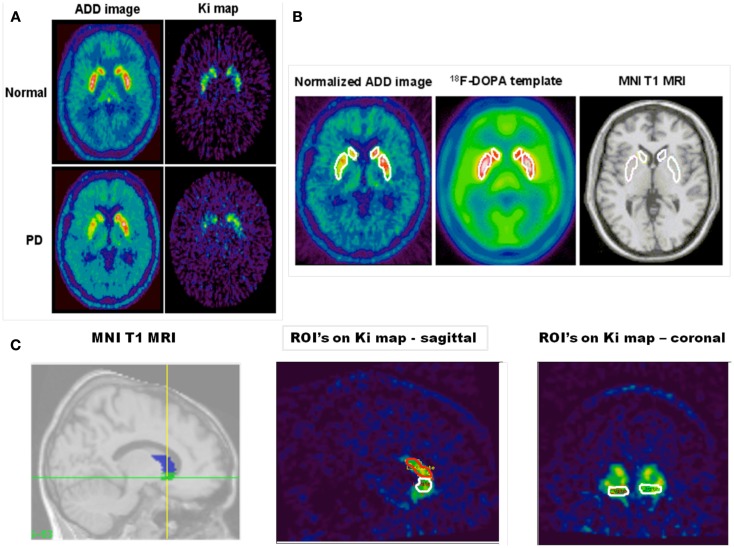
The figure shows in (A) an FDOPA ADD (summed) and net influx rate constant (*K*_I_) image of a single slice from a healthy individual (top panels) and a patient with PD (bottom panels). In these images normalization to (MNI) space has been performed. Asymmetric decreased putamen and caudate uptake can be seen in the patient with PD. In (B) the figure shows; a template region object map overlying: the putamen and caudate nucleus bilaterally in a normalized FDOPA ADD image from a PD patient; the FDOPA template (created from five healthy controls) and the canonical single-subject T1 MRI found in SPM 99. (C) Shows the template object map, with volumes shaded, for the ventral striatum and caudate nucleus overlain on the canonical single-subject T1 MRI in sagittal section and next to it a spatially normalized K_I_ image in sagittal section with the ventral striatum and caudate nucleus object map overlain. Also shown is a spatially normalized coronal section K_I_ map through the ventral striatum with the object map overlain.
